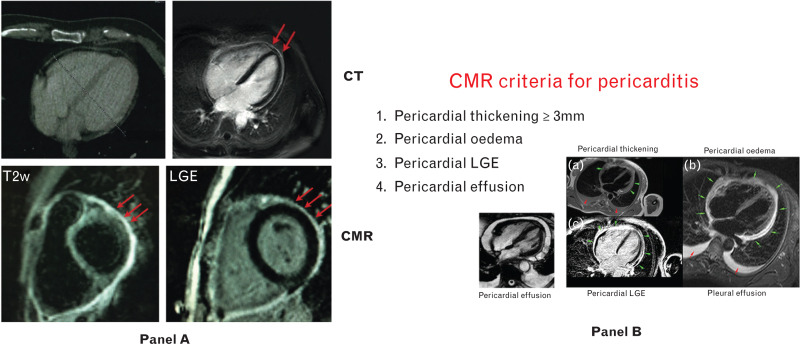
The inflamed pericardium is neovascularized and becomes contrast-enhanced on computed tomography and cardiac magnetic resonance (panel a). Proposed cardiac magnetic resonance (CMR) criteria for pericarditis (panel b).
